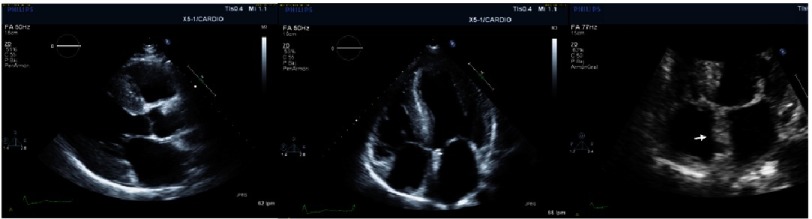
2-dimensional echocardiographic images of a patient with ATTR-CA. Concentric LVH is visible in the first on the left and in the image in the middle. Ground glass appearance of the myocardium is also apparent. In the image on the right hand side we can appreciate interventricular septum thickening (white arrow) and valve thickening.
